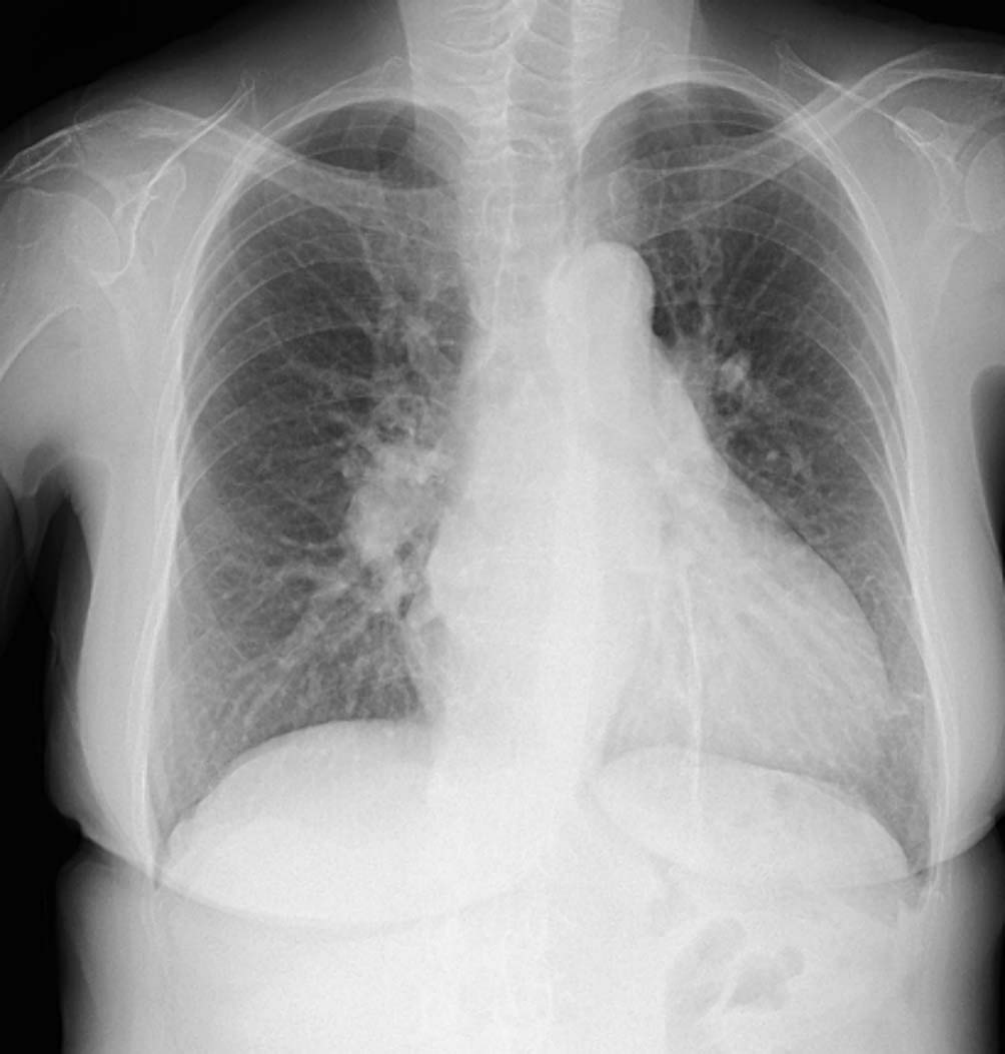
Chest X-ray showing a CTR of 58% and CPA sharp.
